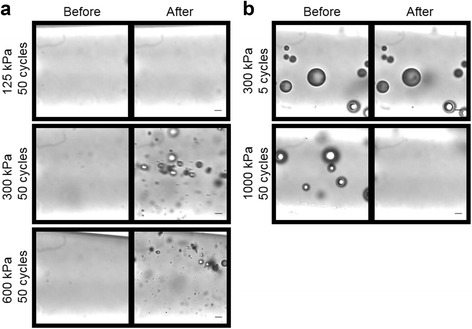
Observation of PCCA vaporization and secondary microbubble effects using high-speed photography. Representative photos are displayed of PCCAs or resultant microbubbles before and after ultrasound stimulation (1.0 MHz center frequency). a The nanoscale, liquid-filled PCCAs are difficult to observe before vaporization. A peak negative pressure of 125 kPa is not sufficient to vaporize the PCCAs (*top*). With a peak negative pressure of 300 kPa, efficient vaporization of the PCCAs into microbubbles is observed (*bottom*). b Secondary effects are observed when generated microbubbles are subjected to a second acoustic pulse. At 300 kPa and 5 cycles, the second acoustic pulse appears to have no affect on the generated microbubbles (*top*). With high acoustic energies, complete microbubble destruction is observed (*bottom*). *Scale bar* = 10 μm. a Vaporization b Secondary effects
